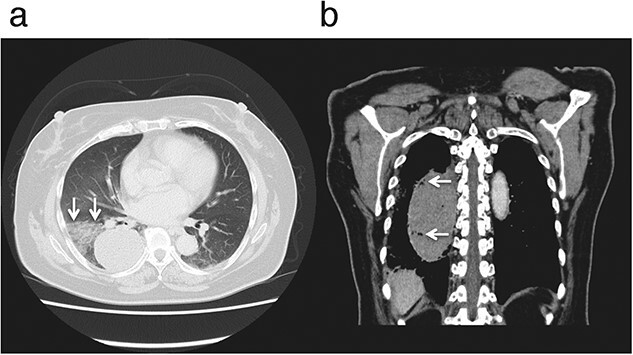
Chest contrast-enhanced computed tomography images of a hemoptysis patient showing a nonenhanced, oval-shaped, well-circumscribed solitary mass in the right lower lobe. (a) Transverse view of the lung window image reveals a surrounding halo of ground-glass opacities (*arrows*). (b) Coronal view of the mediastinal window image displays intramass air bubbles (*arrows*). Both findings indicate the rupture of a hematoma into the lung tissue.
